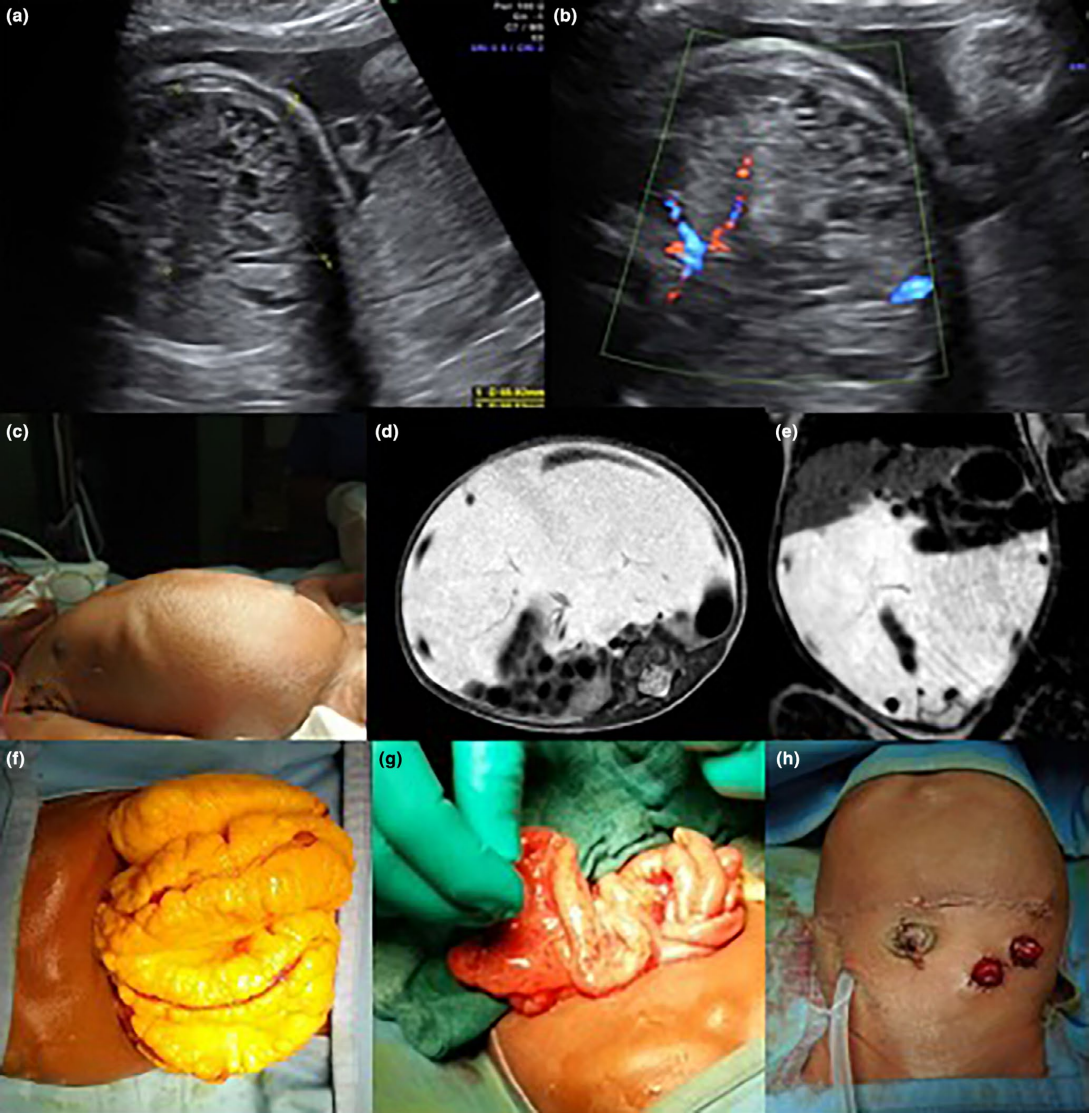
Prenatal diagnosis of a large abdominal mass identified as a lymphangioma, treated surgically. (a, b) Prenatal ultrasound showing an abdominal mass, suspected to be a lymphangioma. (c) Abdominal distension in the newborn at birth. (d, e) Postnatal abdominal magnetic resonance imaging confirms the prenatal suspicion of lymphangioma. (f–h) Laparotomy reveals an extensive intestinal lymphangioma, which is drained and removed with the creation of intestinal stomas.
